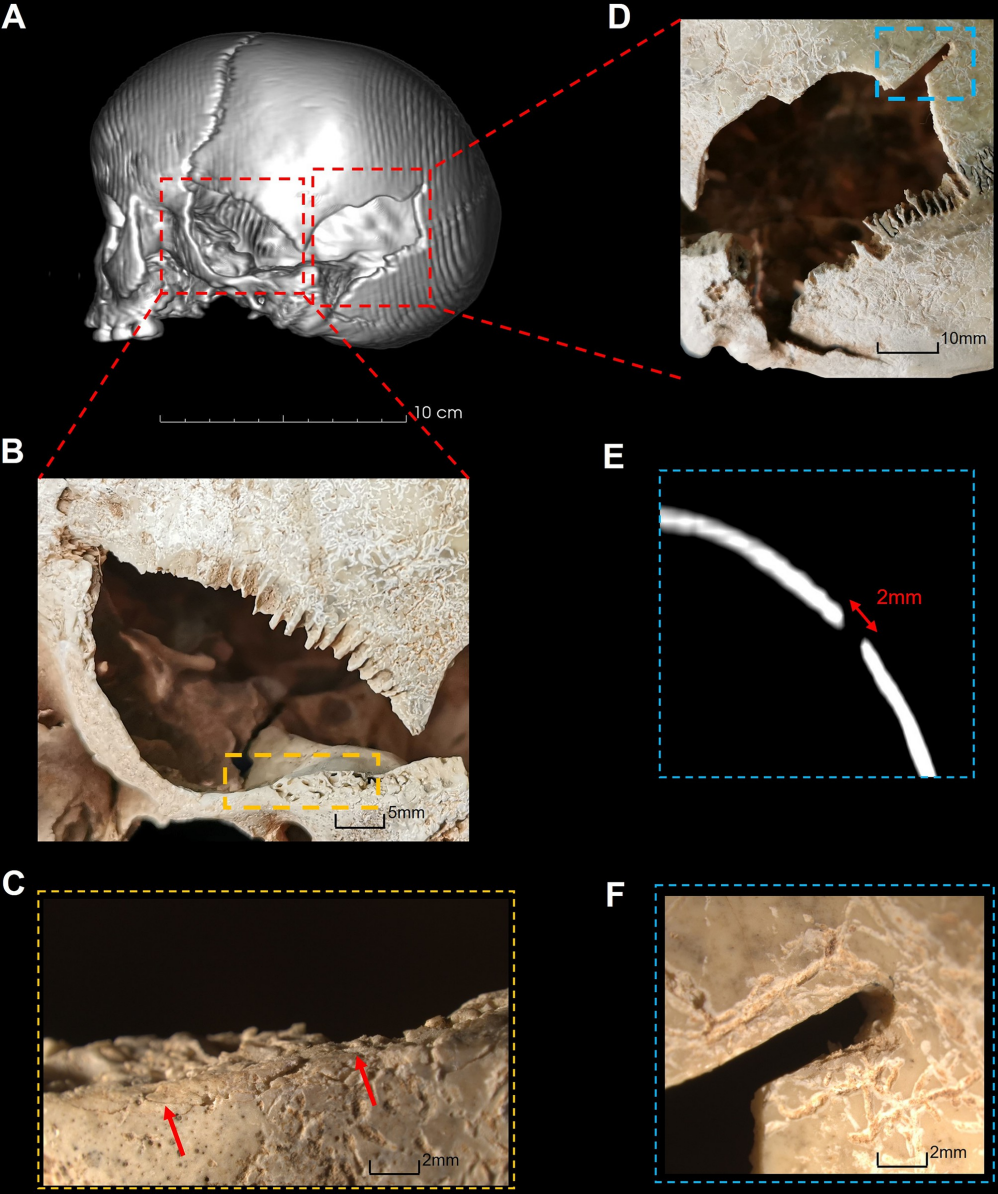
Cranial injuries on UCT 149. A: 3-Dimensional CT rendering of left-posterior cranium. B: Penetrating injury to left temporal region. C: Bone scales on inferior margin of B (red arrows). D: Penetrating injury to left parietal region. Blue box: Linear defect on superoposterior aspect of lesion. E: Measurement of linear defect on axial cross-section CT (2mm). F: Linear defect on superoposterior aspect of D.
